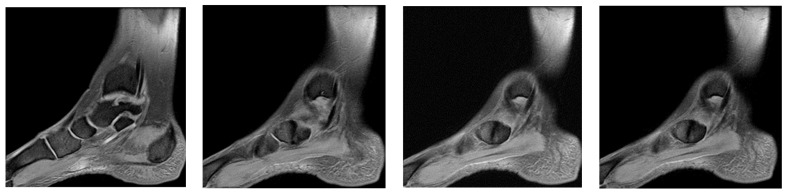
Ankle MRI: sagittal T2 fat sat acquisition.
